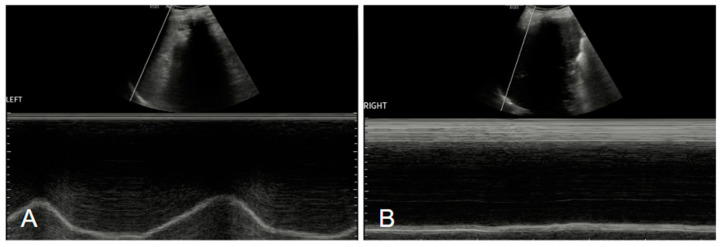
Ultrasound examinations of our patients. The figure shows M-mode studies of the diaphragm, with normal movement of the left hemidiaphragm (A) and impaired movement of the right hemidiaphragm (B) [6].
